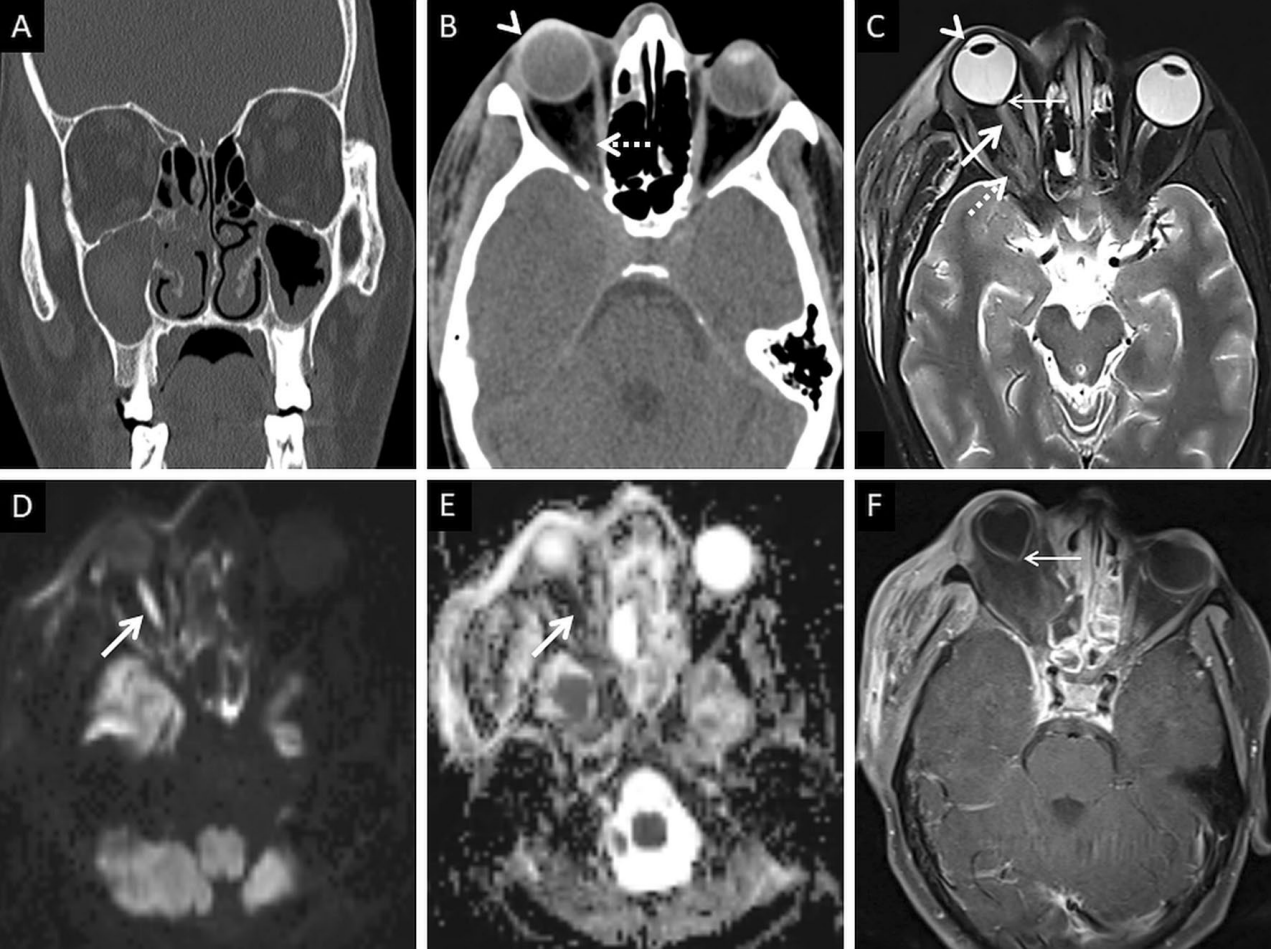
ROCM-related ONI and endophthalmitis. Coronal CT image of the paranasal sinuses (a), obtained using the bone algorithm, reveals opacification of the maxillary sinuses, worse on the right. Axial CT image (b), using the soft tissue algorithm, demonstrates subtle right orbital fat stranding (dotted arrow) and mild right proptosis (arrow head). Axial T2-FS image (c) confirms the right orbital stranding (dotted arrow) and right proptosis (arrow head). Note the subtle tenting of the posterior aspect of the right globe (thin arrow) and thickening of the right optic nerve (thick arrow). Axial diffusion weighted (d) and apparent diffusion coefficient (e) images reveal restricted diffusion within the right optic nerve in keeping with an optic nerve infarction. Overall, findings of orbital compartment syndrome with endophthalmitis and optic nerve infarction was suggested. The patient underwent urgent surgery, with removal of the right lamina papyracae, for relief of the compartment syndrome. CE FS axial T1W image (f) reveals interim worsening of the right endophthalmitis (thin arrow)
